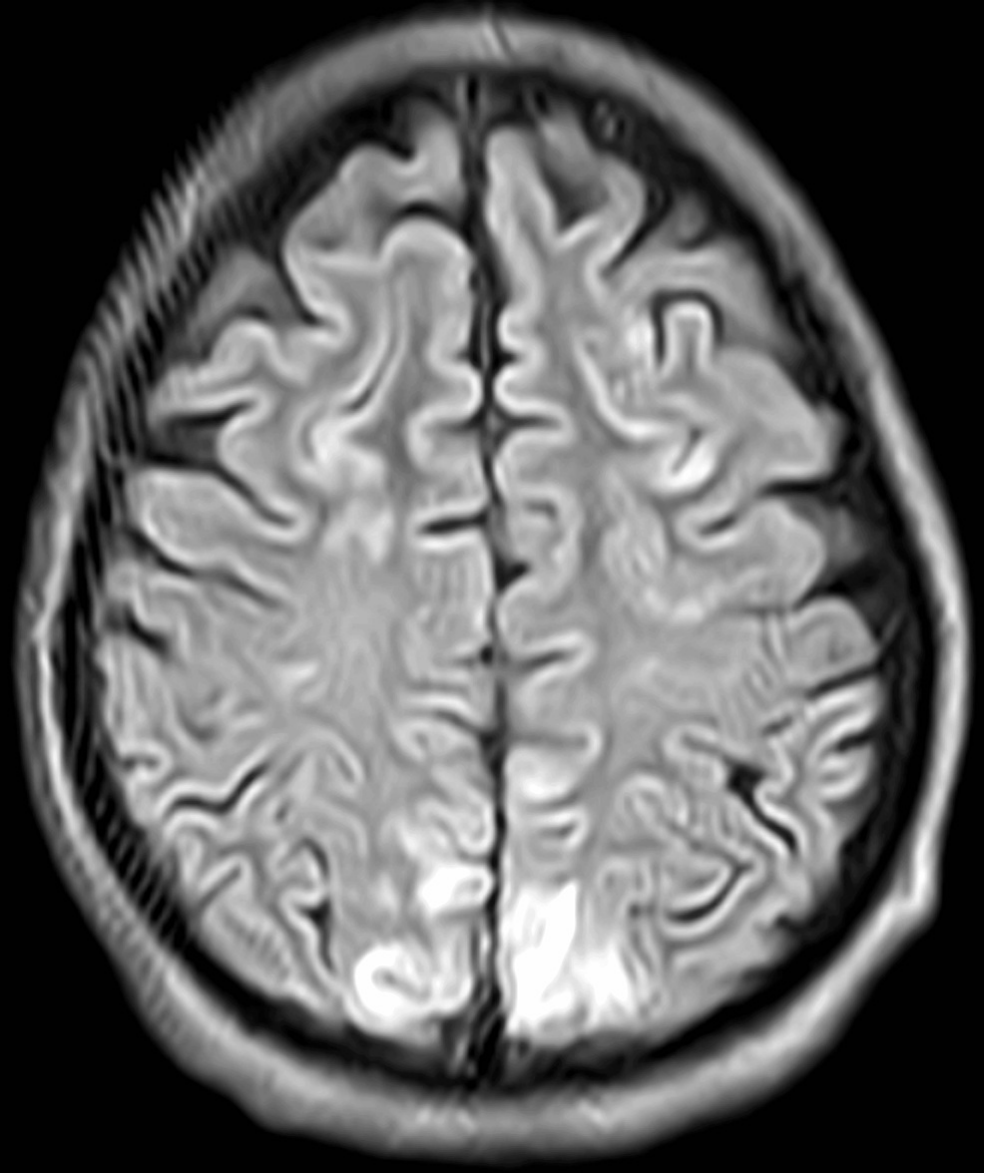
Diffusion restriction involving the occipital lobe on MRI fluid-attenuated inversion recovery.
